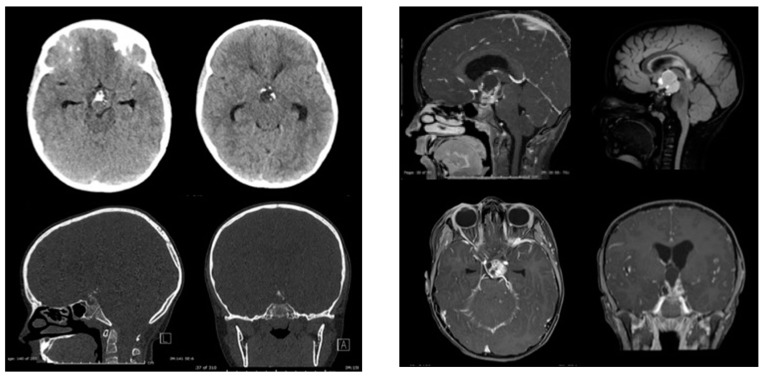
Computed Tomography (Left) andMagnetic resonance Imaging (Right) images from the same patient of a classic example of an adamantinomatous craniopharyngioma. This typical tumor is centered in the suprasellar region with mixed solid and cystic areas as well as areas of calcification as seen on the CT.
